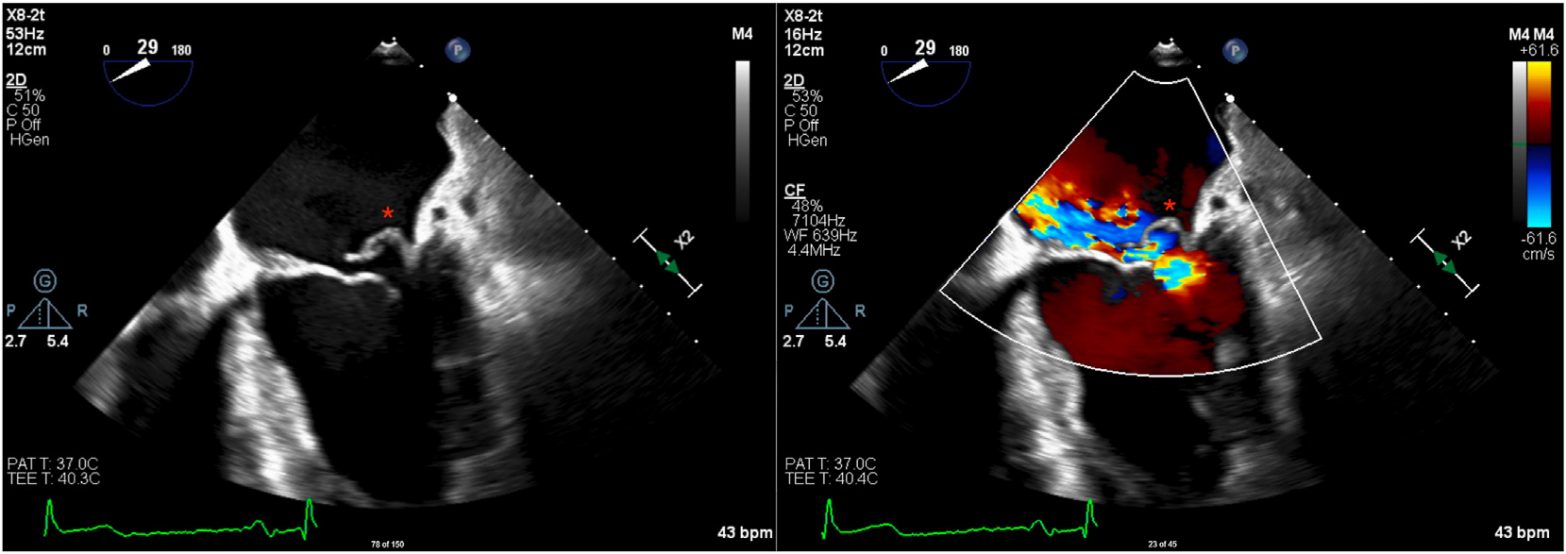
Two-dimensional TEE, midesophageal four-chamber (29°) view, systolic phase without (*left*) and with (*right*) color flow Doppler, demonstrates the posterior flail leaflet (*asterisk*) and very eccentric, anteriorly directed MR jet.
